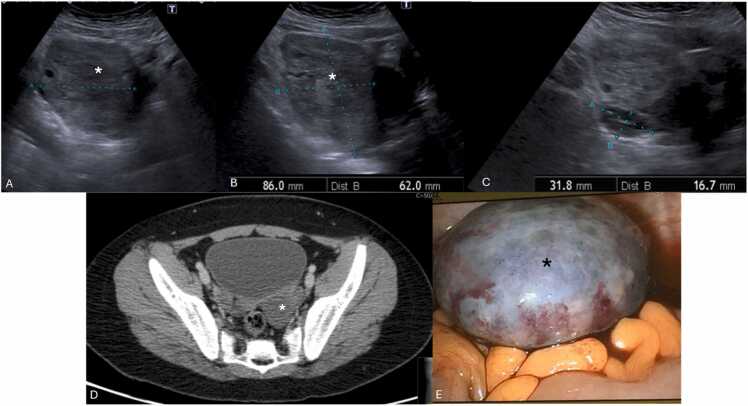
Suprapubic pelvic ultrasound in (A–C) in a 13-year-old female, presenting with sudden pelvic pain, revealed an asymmetrically enlarged left ovary with a volume > 200 cc, with heterogeneous echotexture and loss of normal follicles (* on A and B). Compare with the volume of a normal right ovary (between calipers in C). Due to diagnostic uncertainty, contrast-enhanced pelvic CT (D) was obtained, which shows the enlarged, heterogenous, left ovary in an abnormal position (* in D), with absent central enhancement after contrast administration. Due to clinical and radiological suspicion of ovarian torsion, the patient underwent emergent laparoscopy, and during surgery, a necrotized ovary (* in E) was noted and photographed.
